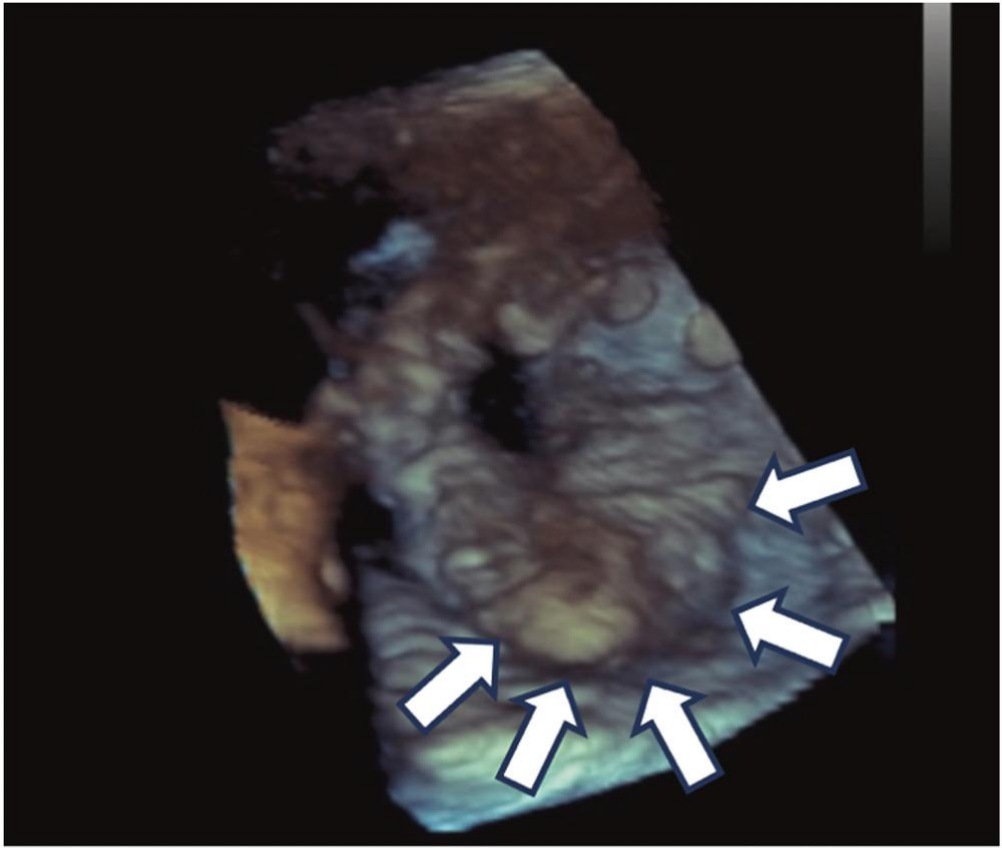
Transoesophageal echocardiography of obstructive bioprosthetic valve thrombosis. 3D echocardiographic images of the bioprosthetic valve after surgical aortic valve replacement. A thrombus is present on the prosthetic valve, restricting its motion (white arrows).
